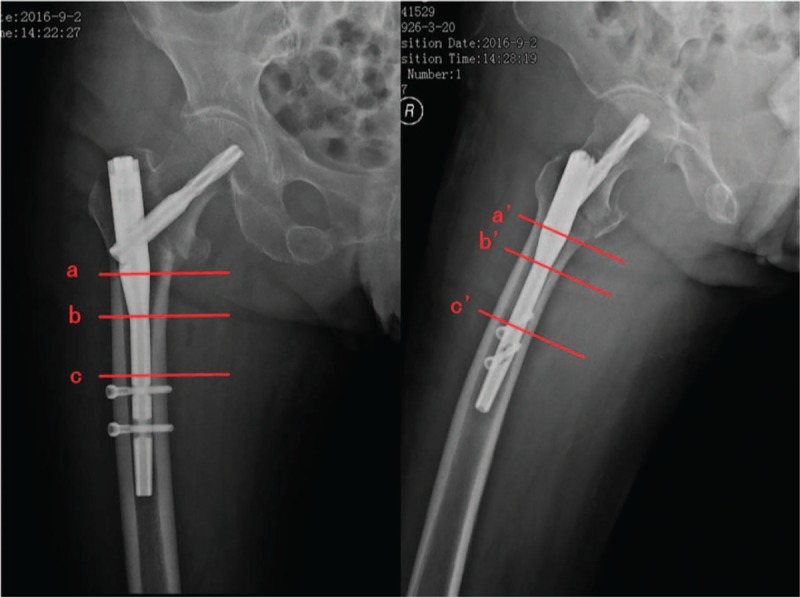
(A) Frontal and (B) lateral X-ray images of intertrochanteric fractures. a and a’, start of funnel; b and b’, end of funnel; c and c’, femoral isthmus.
